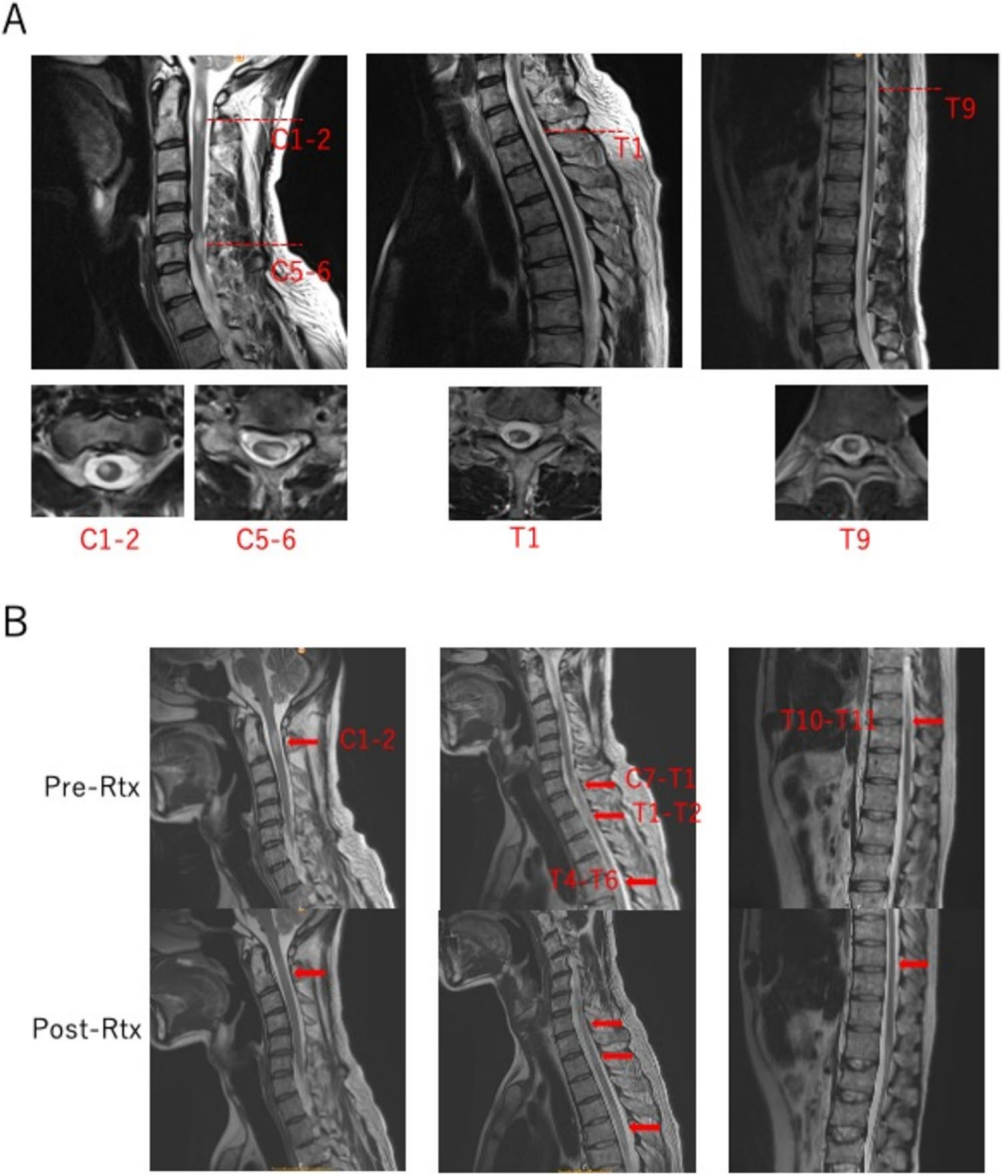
T2 weighted images of MRI a Abnormal lesions at the onset. High-intensity lesion observed at C1-2, C5-6, T1, and T9 levels. b Changes of abnormal signals at C1-C2, C7-T1, T1-T2, T4-T6, and T10-T11 levels pre and post rituximab treatment. Each abnormal signal of lesions attenuated after rituximab treatment
